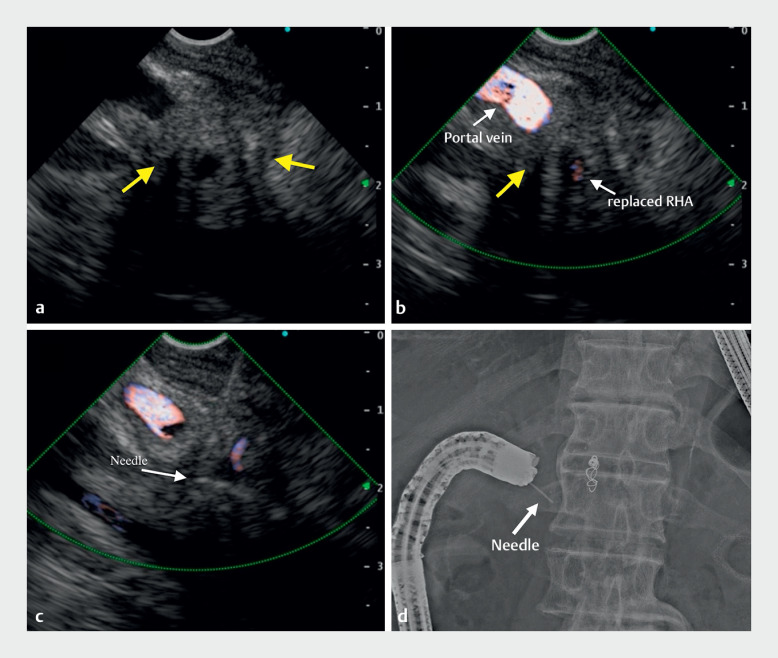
Endoscopic ultrasound-guided tissue acquisition.
